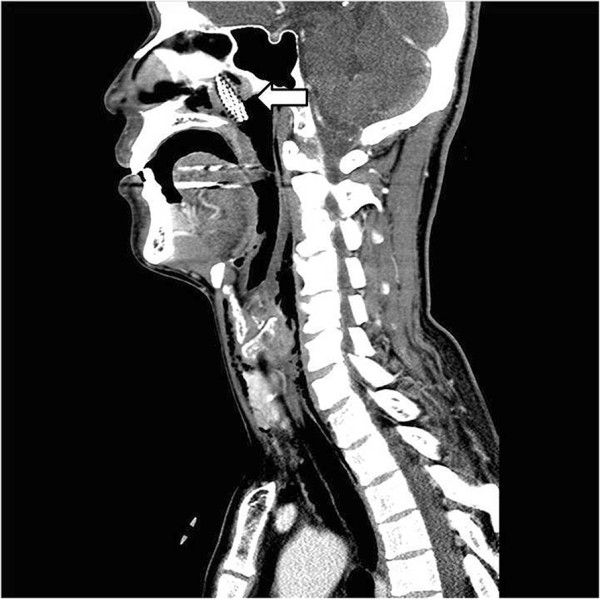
Sagittal view of neck CT scan. (Arrow) A neck CT shows the toothbrush spanning the nasopharynx and oropharynx, as well as subcutaneous emphysema in the anterior and lateral cervical regions.
